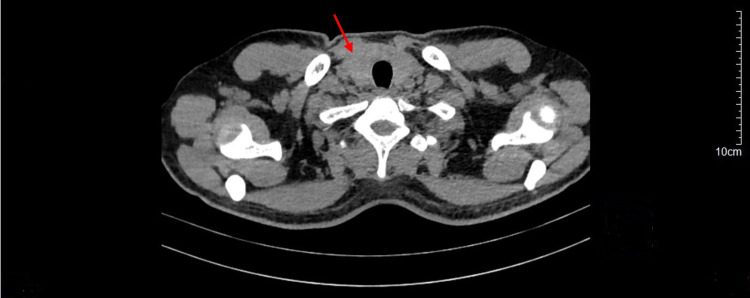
Axial View of Chest Non-Contrast CT Scan Showing Enlarged Right Thyroid Lobe (Red Arrow)
